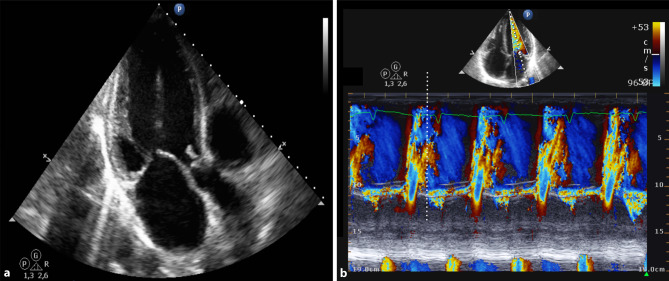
a Still frame from apical three-chamber view; b Colour M‑mode of transmitral flow from the apical four-chamber view
